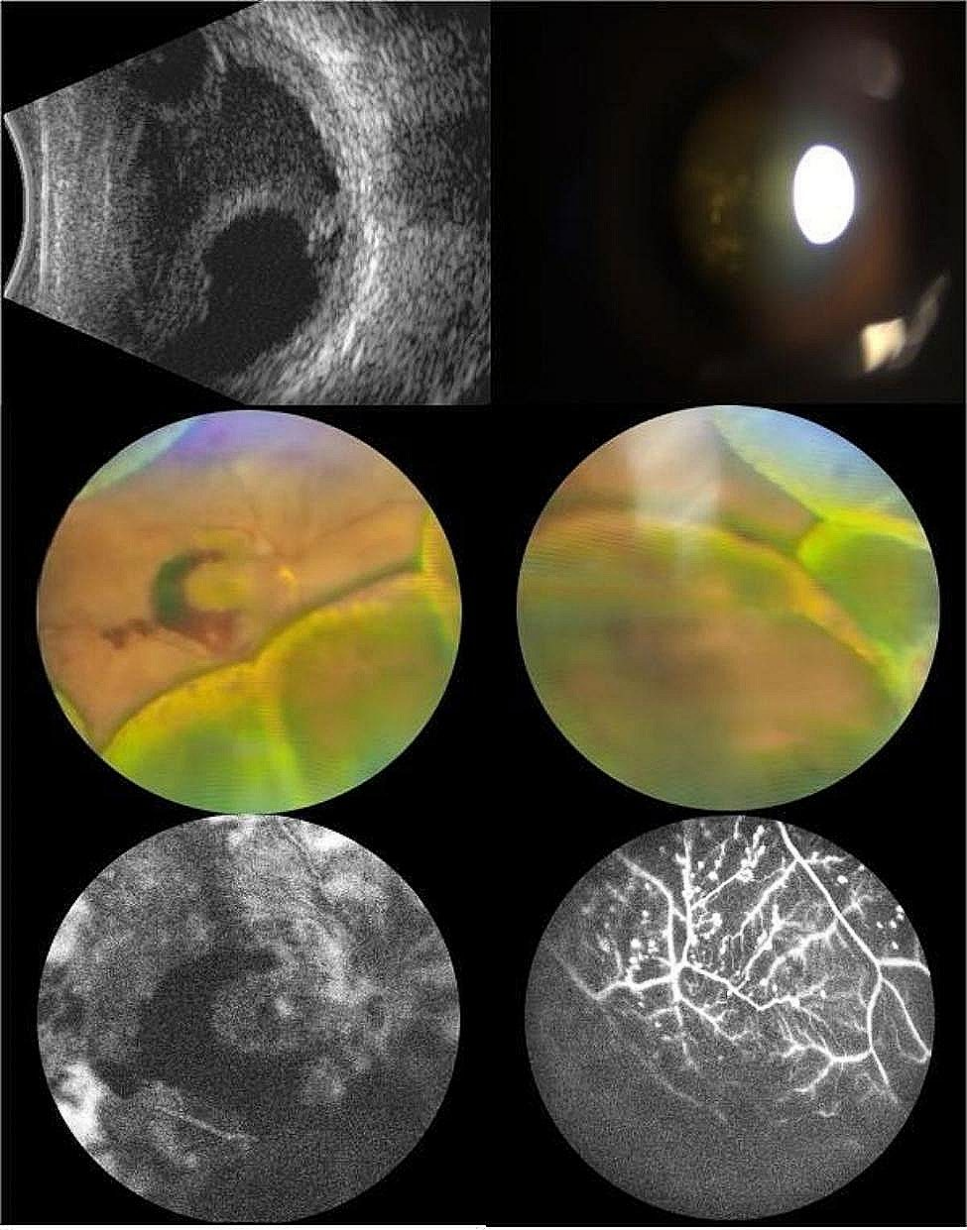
Eye condition of the patient on admission. a: B ultrasound: vitreous opacity, choroidal detachment and retinal detachment in both eyes. b: Glass white pompon-like cloudy. c: Fundus photography of the right eye. d: Fundus photography of the right eye. e: posterior pole FFA. f: peripheral imeter FFA
